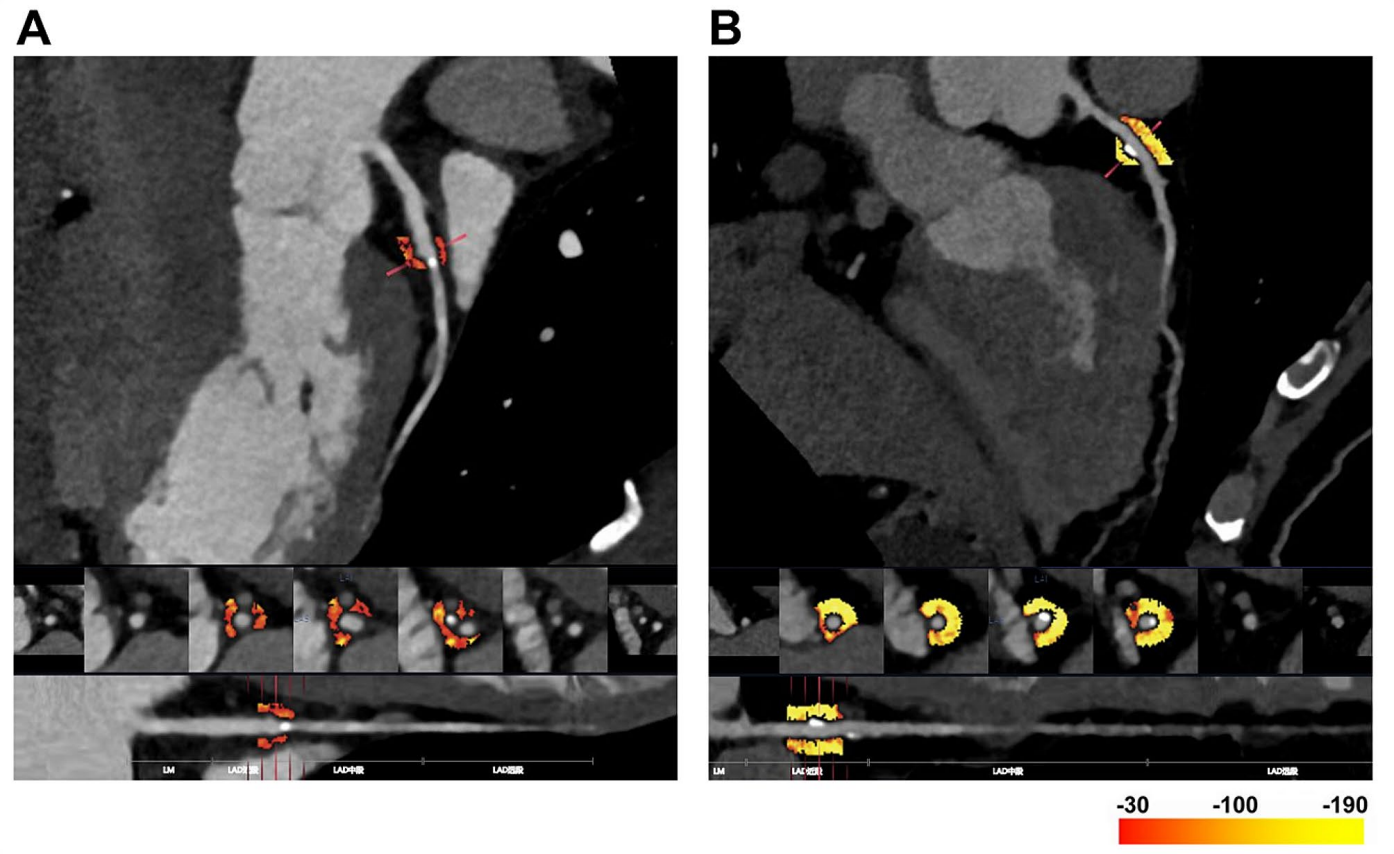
Two typical cases of lesion-specific pericoronary FAI differences between patients with and without MACE measured by CCTA. A A 59-year-old patient (male) with MACE, the lesion-specific pericoronary FAI was − 60HU. B A 61-year-old patient (male) without MACE, the lesion-specific pericoronary FAI was − 110HU. *FAI* fat attenuation index, *MACE* major adverse cardiovascular event, *CCTA* coronary computed tomography angiography, *HU* Hounsfield Unit
